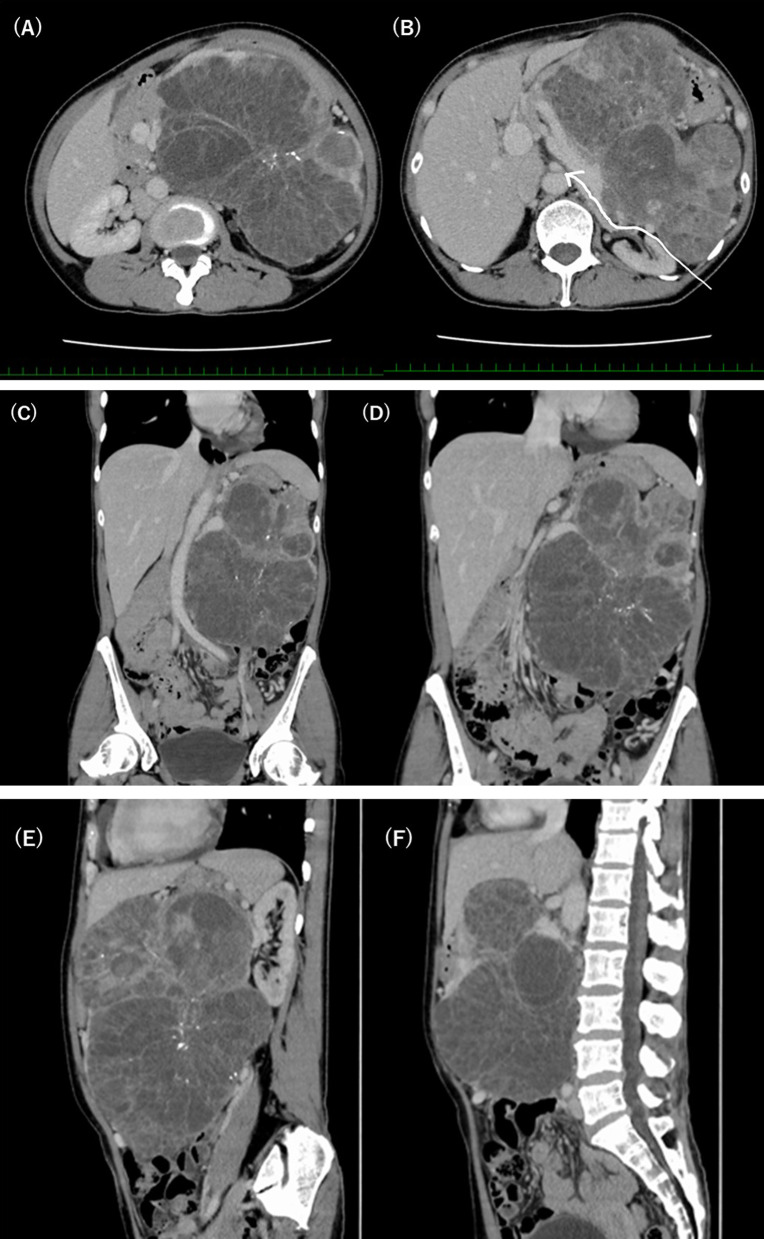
A A typical low-density multi-cystic lesion of SCN at the pancreatic tail was revealed by contrast-enhanced CT at porto-venous phase. B Tumor dorsally compressed the splenic vein, left renal vessels, and left kidney. The white line indicated the estimated dissected space at the dorsal dissection. C Coronary image showed the tumor compression of liver, spleen, abdominal aorta, and left iliac artery. D Tumor compressed the superior mesenteric vessels, and intestines. E Tumor expanded to both the front of the abdomen and the retroperitoneal space. F Tumor spread beyond the level of the abdominal aorta and adjacent vertebra
